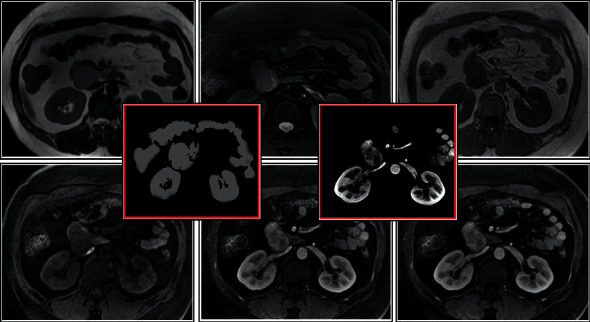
The segmentation effect of the ileus MRI image.
